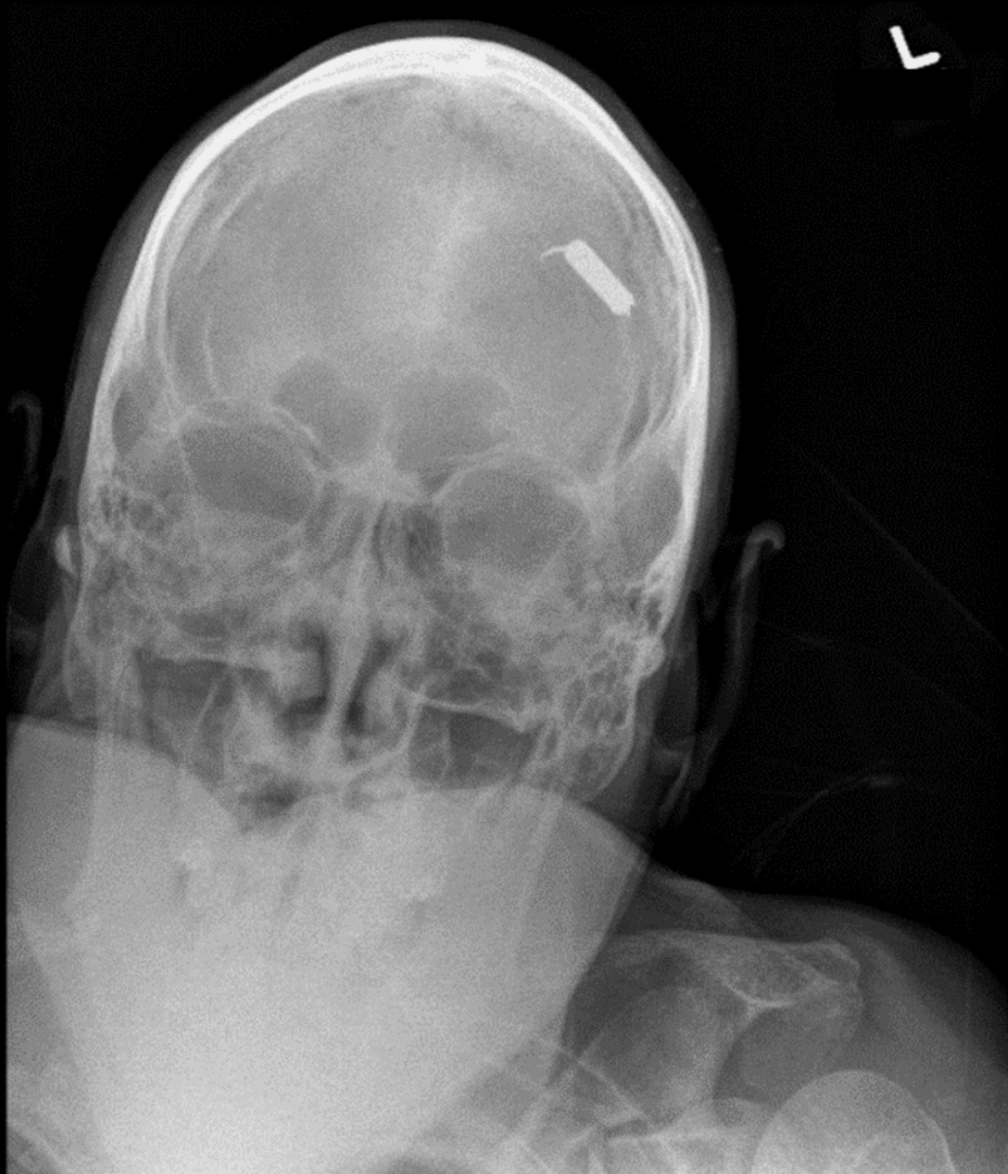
Radiograph of the skull displaying taser barb projecting over the left frontal bone
